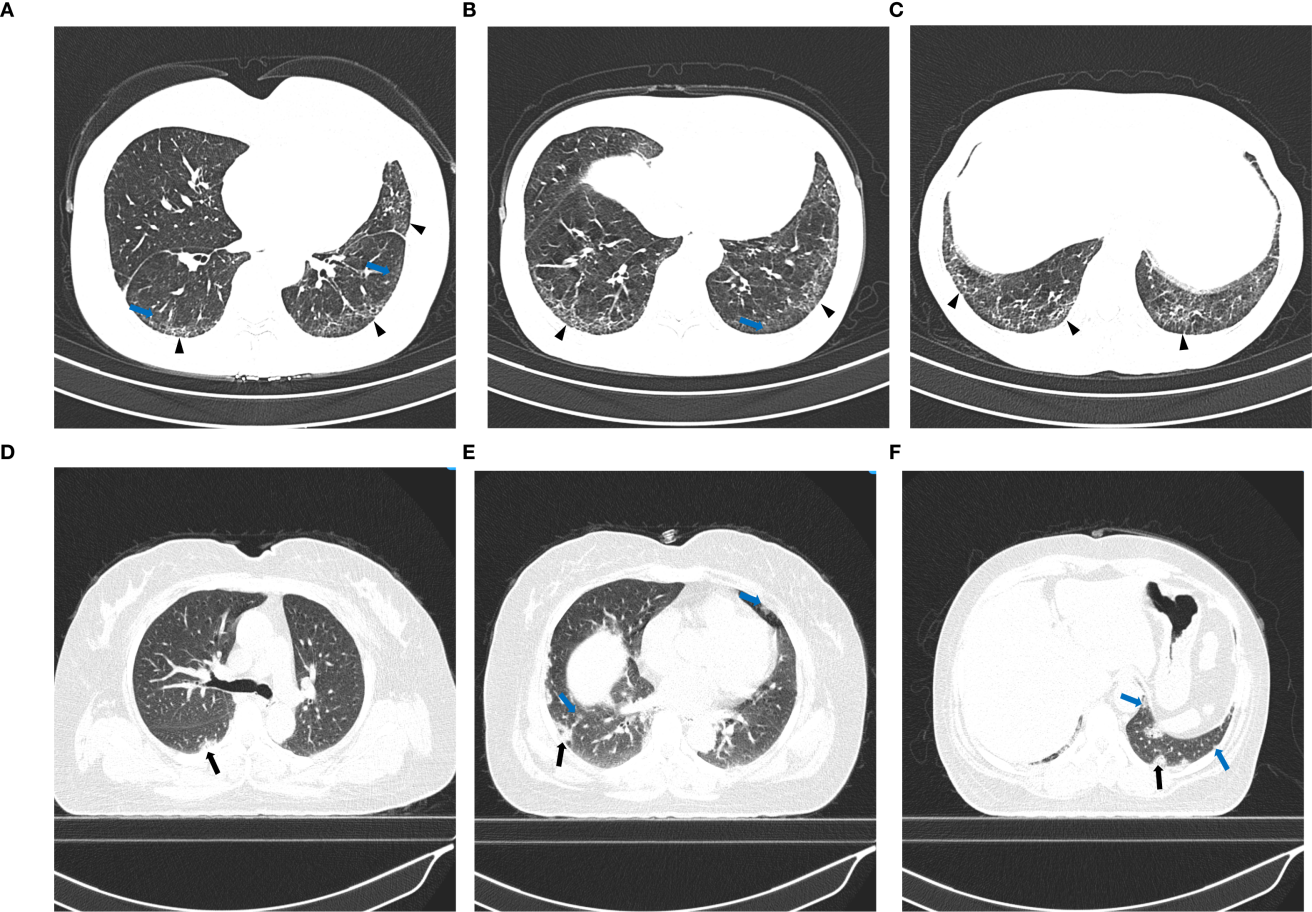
Representative HRCT findings in two patients with PM/DM-ILD. (A–C) A 58-year-old man showing an NSIP pattern with ground-glass opacities involving five lung segments and reticular opacities involving seven segments, with a total HRCT score of 9. Blue arrows indicate ground-glass opacities, and black short arrows indicate reticulation. (D–F) A 49-year-old woman showing an OP pattern with ground-glass opacities involving five lung segments and consolidation involving five segments, with a total HRCT score of 7. Black arrows indicate consolidation.
